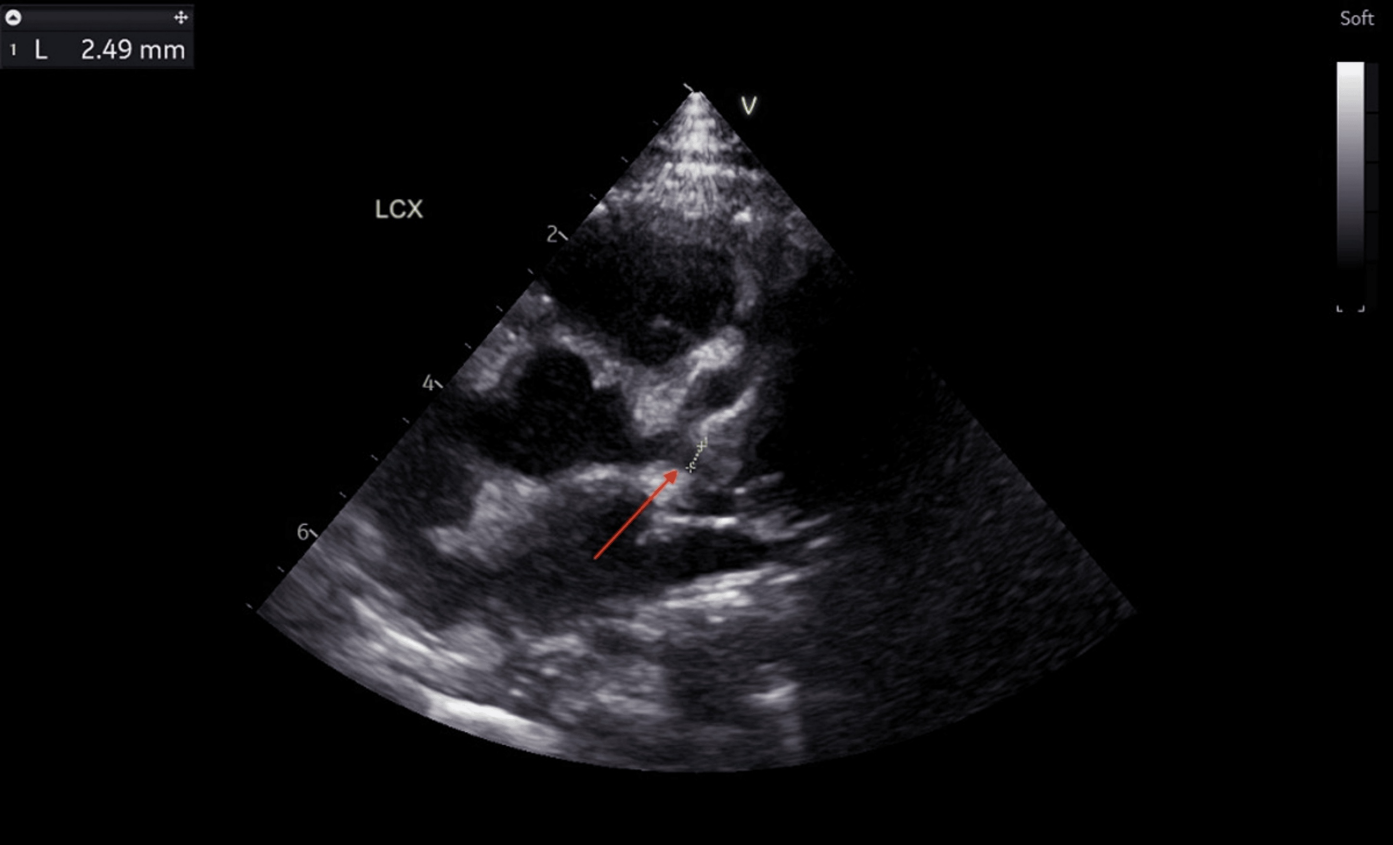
Echocardiogram showing reduction in the left circumflex artery dilatation. The red arrow points at the dilated left circumflex artery measuring 2.49 mm (Z score: +3.60).
